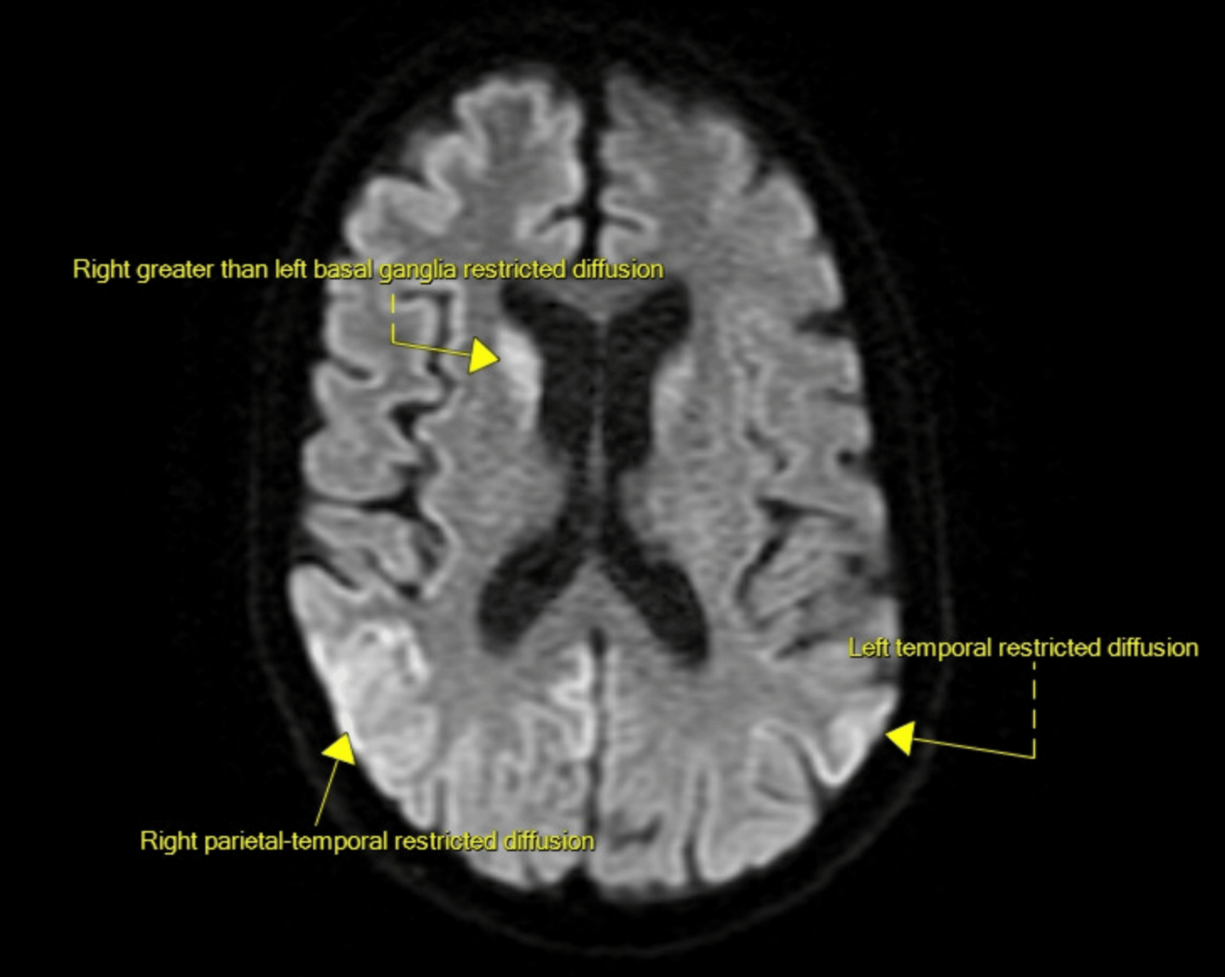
MRI brain with multifocal cortical diffusion signal abnormality and restricted diffusion of the right greater than left basal ganglia
